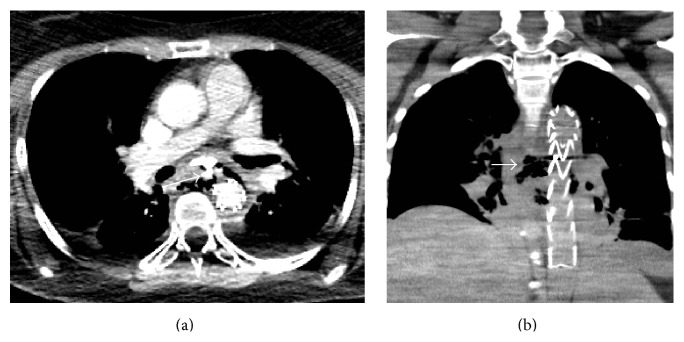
CT scan using oral hydrosoluble contrast material: (a) axial and (b) coronal reconstruction. (a) There is leakage of the contrast material from the esophagus to the posterior mediastinum (white arrow); the aortic stent was placed demonstrating that the esophageal wall is perforated. (b) Mediastinal collection with air bubbles within it (white arrow), surrounding the aorta with the stent placed in an adequate position.
